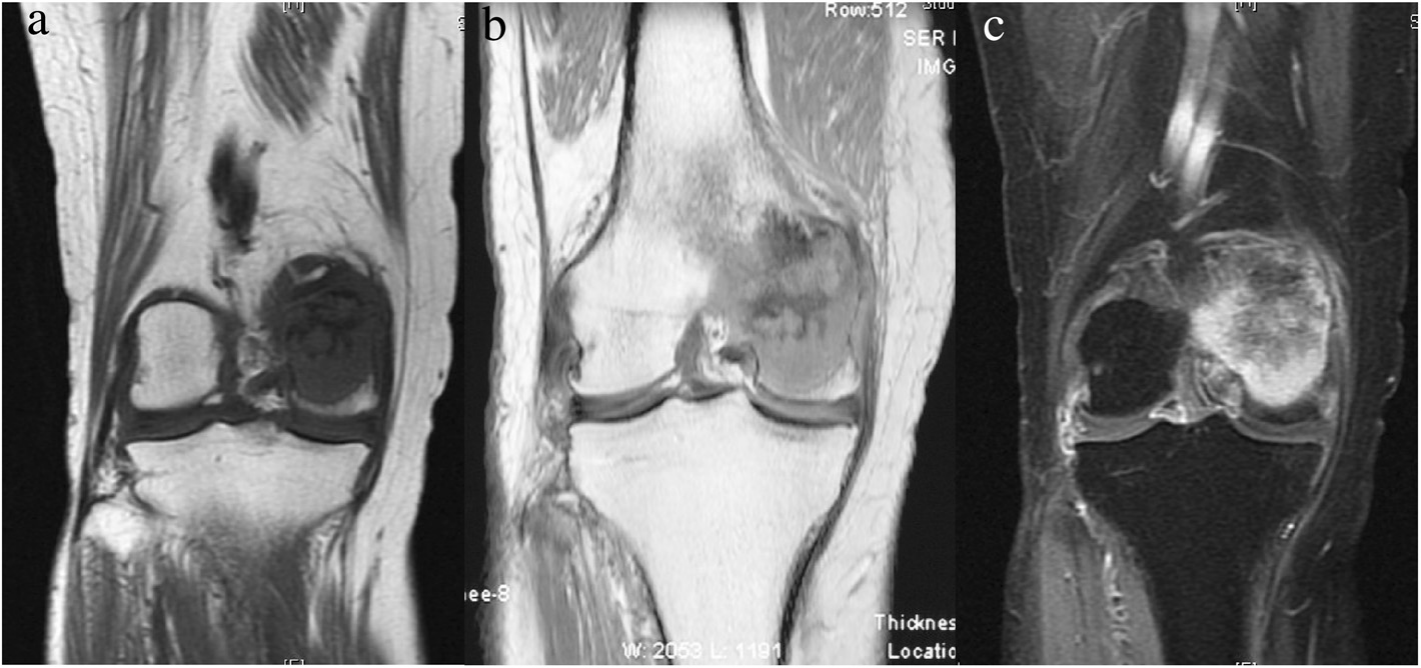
Magnetic resonance imaging of the tumor in the distal femur. On magnetic resonance imaging, the tumor was depicted as a low signal lesion on T1-weighted image (a), low to intermediate intensity on T2-weighted image (b), and highly enhanced with gadolinium contrast (c). There was no apparent destruction of the cortex and no soft tissue mass was present
